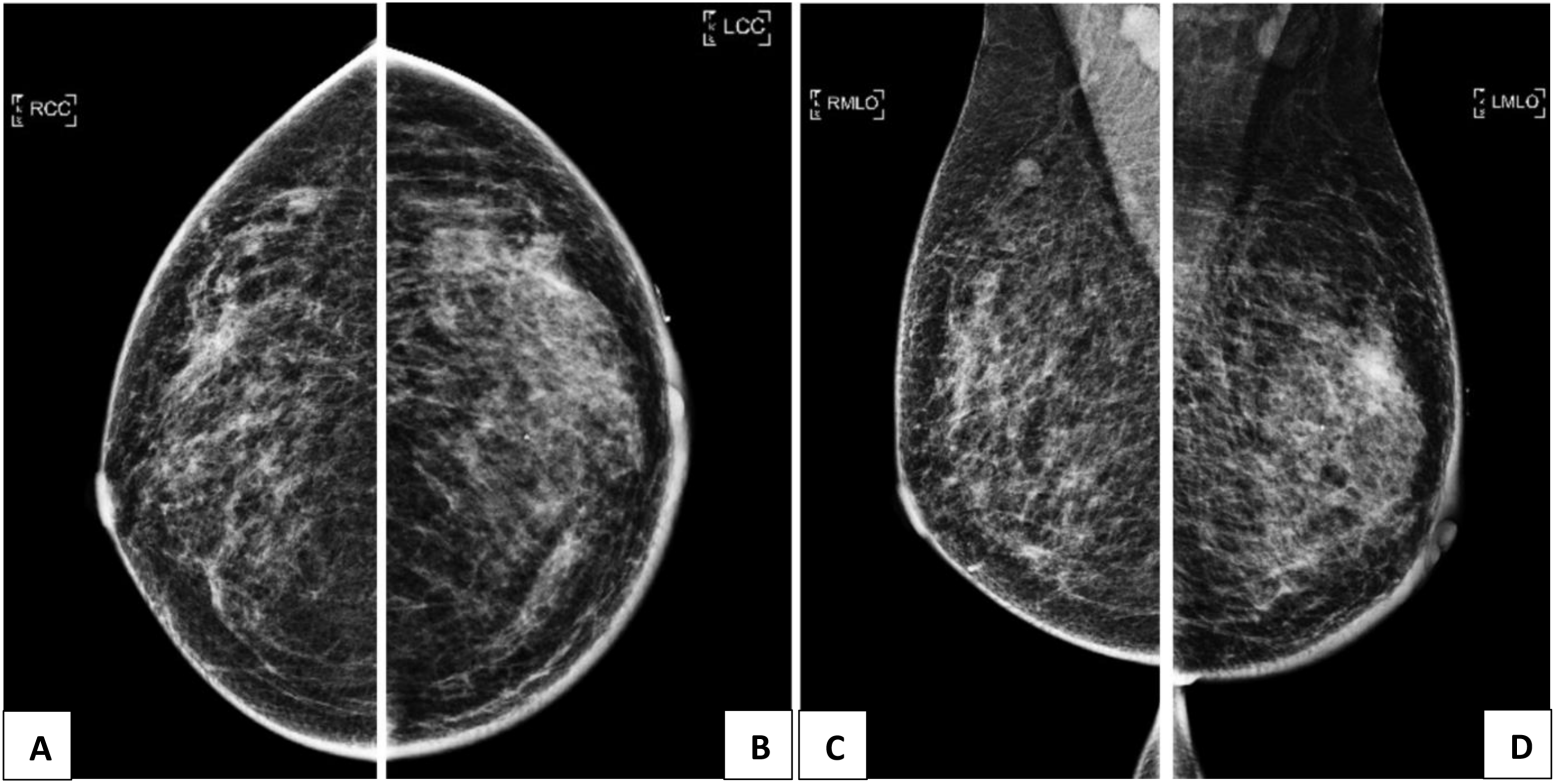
(A, C): Mammogram of the right breast showing an 8 x 8mm partly circumscribed nodule in the right axillary tail, corresponding to the intramammary lymph mode on ultrasound. Abnormal lymph nodes are also seen in bilateral axillae, denser on the right. (B, D): Mammogram of the left breast showing a vague asymmetric density in the upper outer quadrant, corresponding to the palpable lump. The overlying skin is thickened with slight retraction of the nipple.
